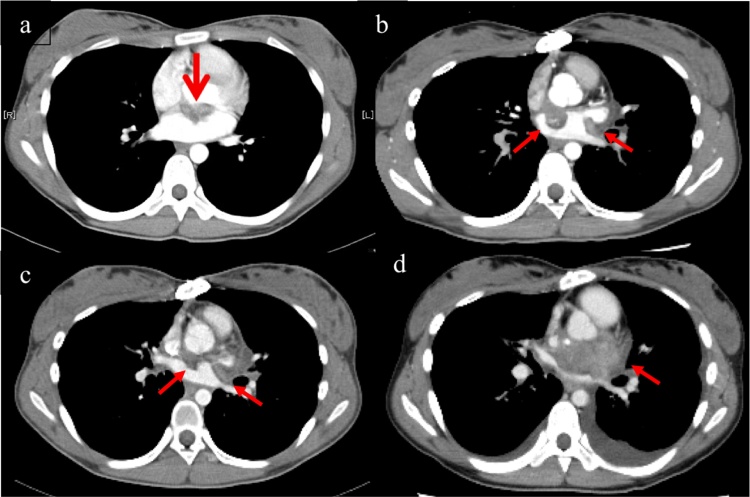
CT images. The tumor gradually grew (Tumor shown as red arrows). (a) Preoperative. (b) 1 year after surgery. (c) 2 year after surgery. (d) 4.5 year after surgery.
